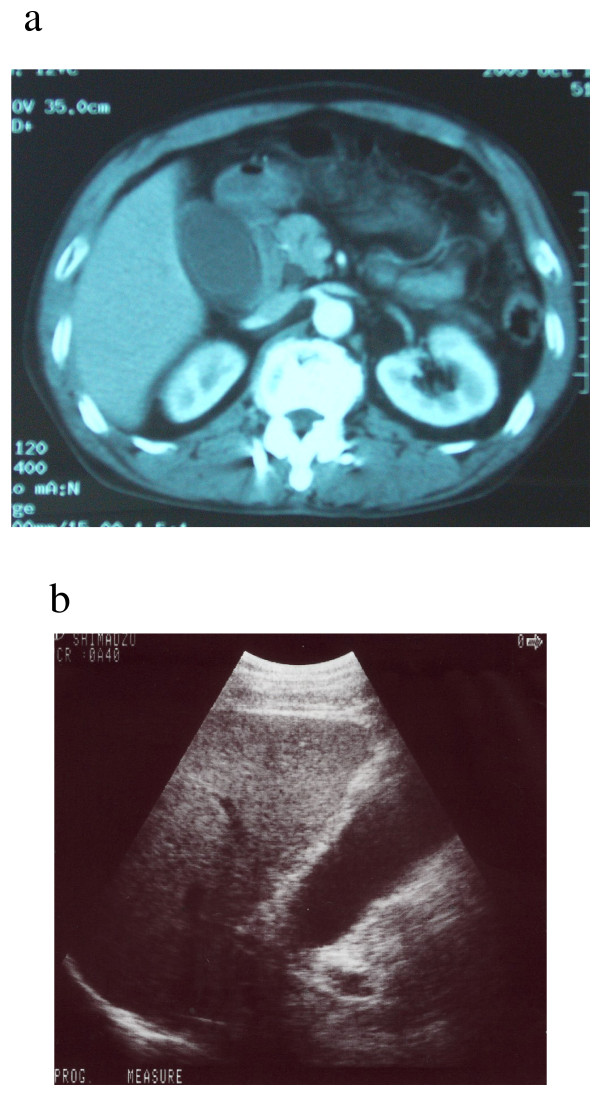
Preoperative imaging findings. Preoperative computed tomography (a) and echography (b) show distention of the gallbladder and thickening of its wall.
